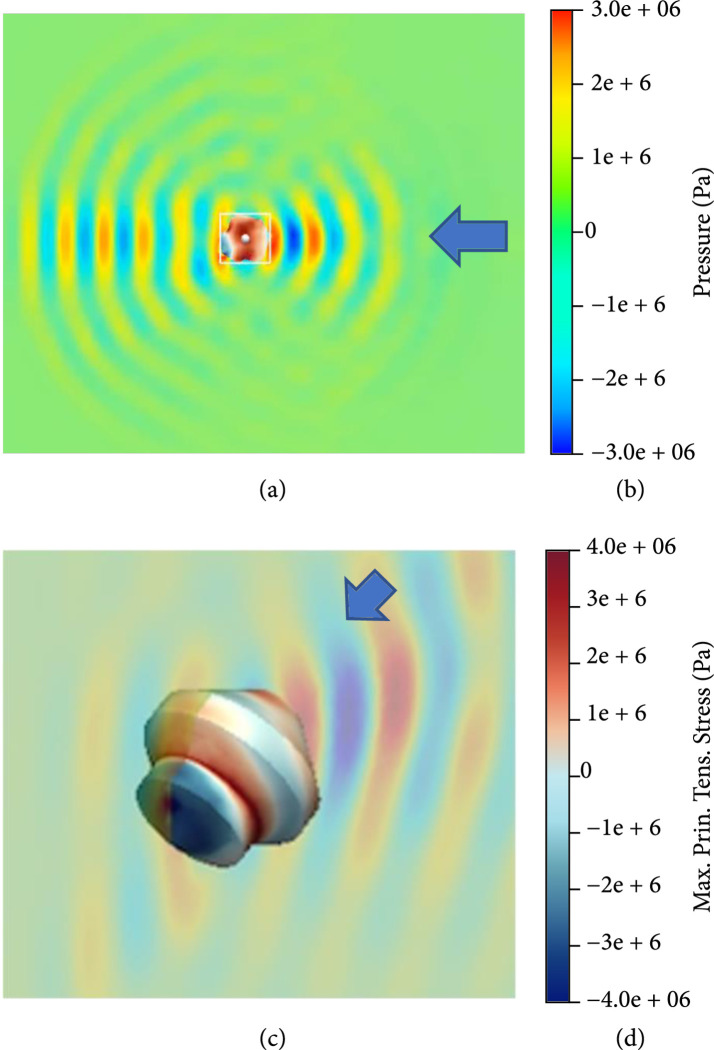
Calculated stress within an irregular 3D stone shape. The axial slice of pressure in the surrounding fluid and stress in the stone ((a) 2.6 mm) is shown zoomed in 3D in (c). The scales (b, d) related to both figures. Arrows indicate the direction of ultrasound propagation (350 kHz). The pressure applied is amplified in the stone, here approximately two times. Above a threshold frequency for a given stone size, amplification is greater than 5 [13] (courtesy of Shunxiang Cao and Tim Colonius, Caltech).
